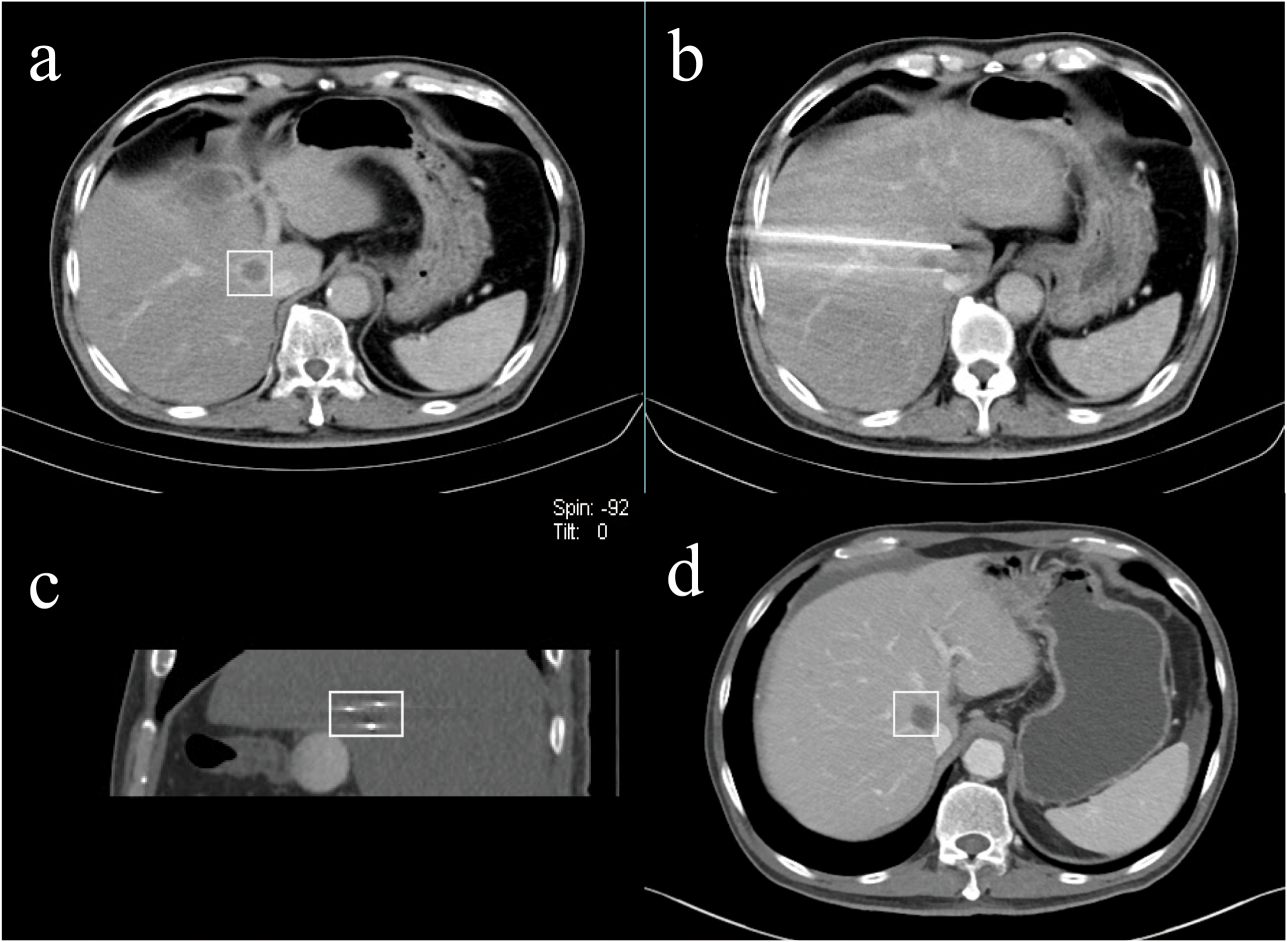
A 66-year-old male with liver metastasis of pancreatic cancer adjacent to the superior vena cava underwent IRE ablation. [(A) venous phase of preoperative enhanced CT (rectangular); (B, C) the approximate equilateral triangle distribution of electrodes; (D) venous phase of postoperative enhanced CT at the 13th-month follow-up indicated satisfactory ablation effect (rectangular)].
